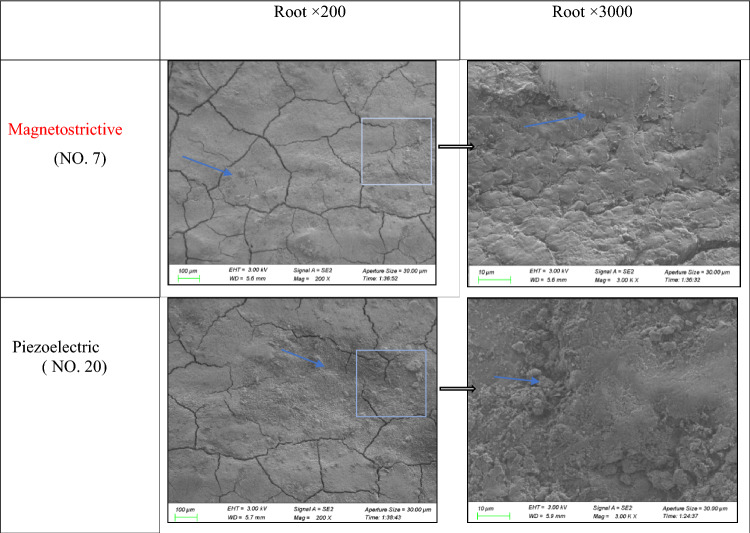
SEM images of root surfaces scaled with two different ultrasonic devices; some smear layers were left on the tooth surface (calculus and smear, layers 3000 ×  →  cementum exfoliation 3000 ×). There was more lost dental material and more smears retained in the piezoelectric group than in the magnetostrictive group.
